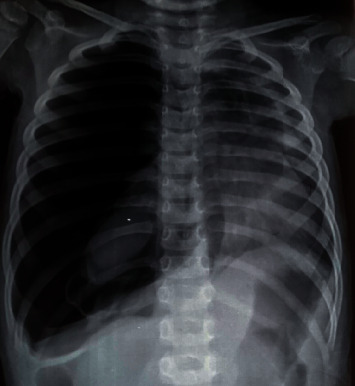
Chest X-ray showing the presence of a right-sided spontaneous pneumothorax with a midline shift to the left.
